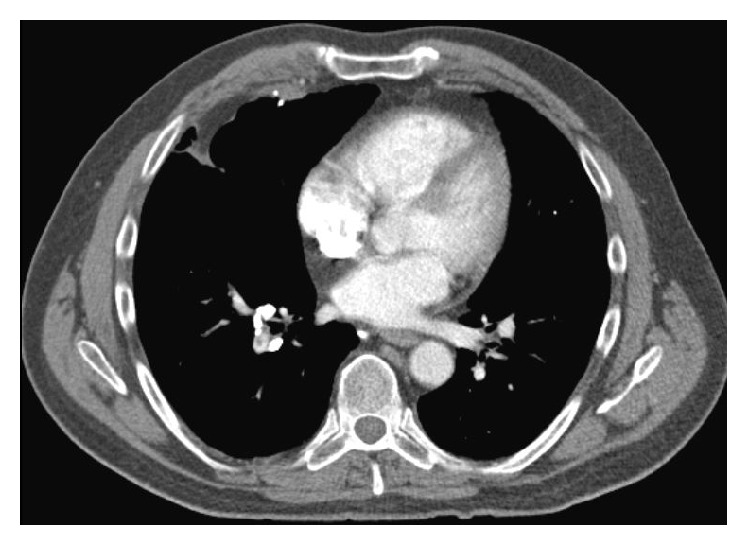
Computed tomography of the chest depicting filling defects in the pulmonary arterial vasculature secondary to pulmonary embolism and probable right middle lobe infarction.
